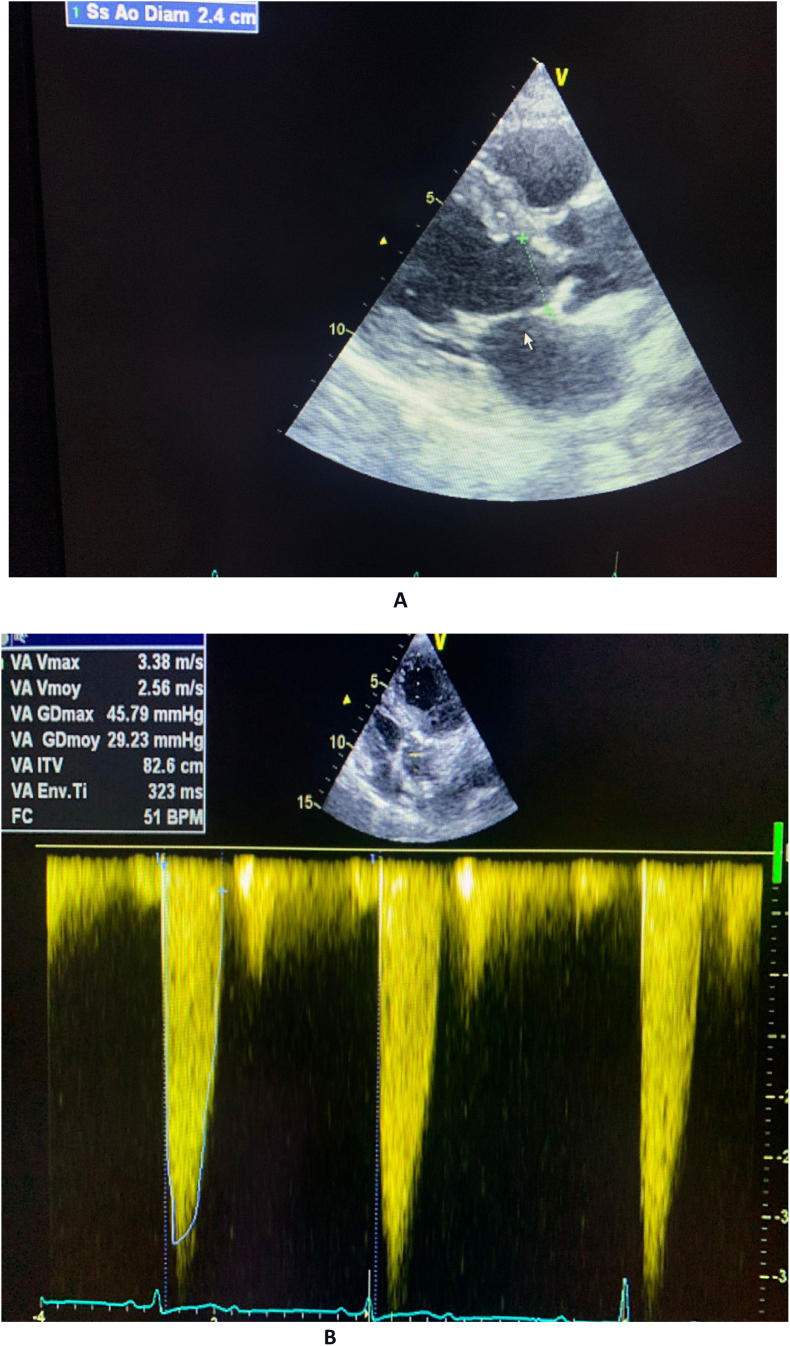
A, B: Baseline echocardiogram: Preserved LV function with moderate aortic stenosis.
